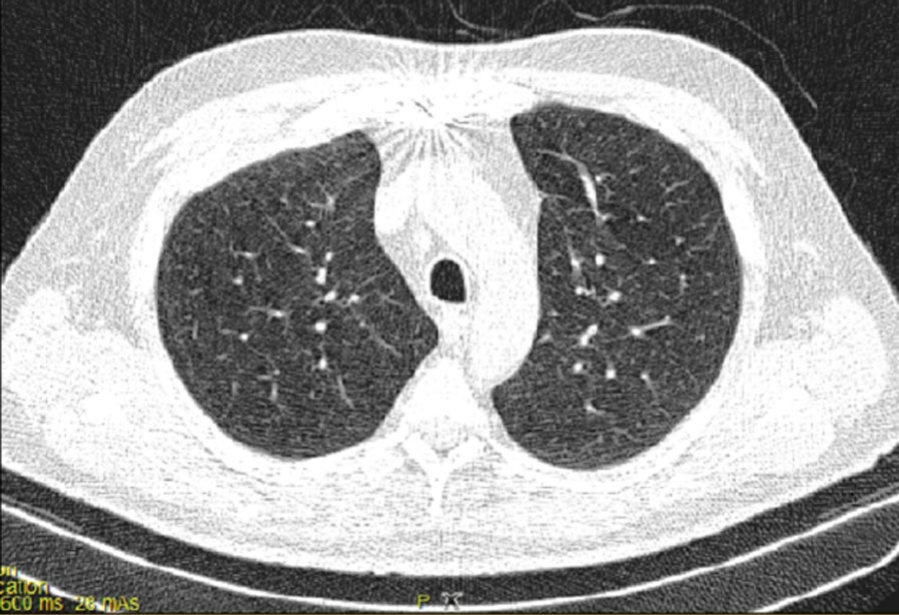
CT image of the lungs, axillary view, showing recovery after treatment
